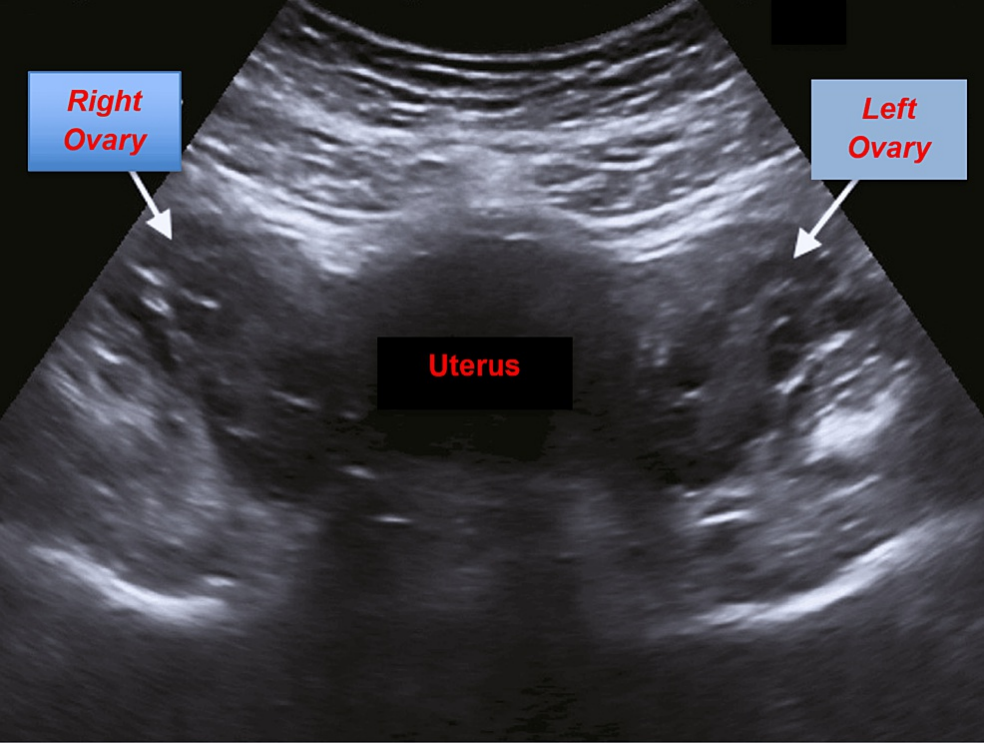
Ultrasound of the pelvis negative for any ovarian mass or cyst
